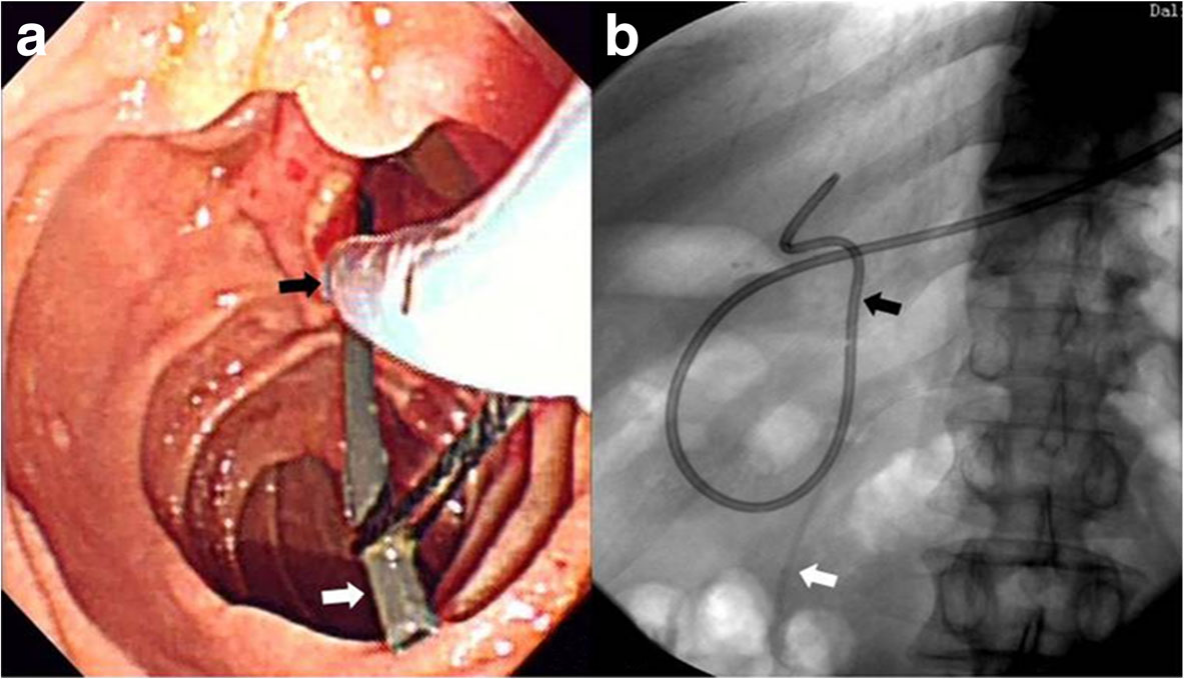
Physical map of type three pancreatic stent: the white arrow indicates the pancreatic stent. The black arrow indicates the nasobiliary catheter. a An endoscopic image that shows the pancreatic stent placement in the pancreatic duct. b An X-ray image that shows the nasobiliary catheter in common bile duct and the PS linked together by the line
